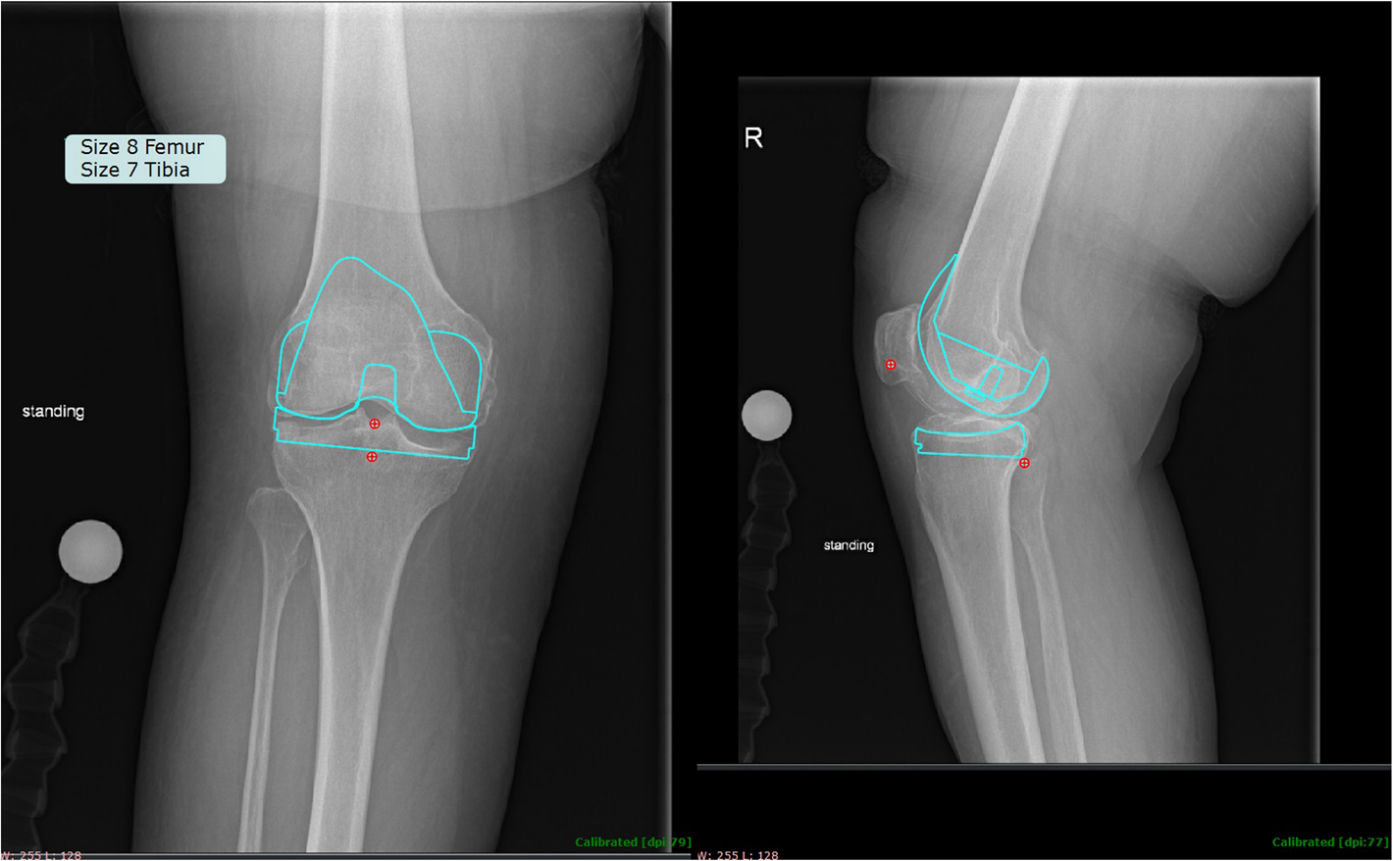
Example case where demographic information outperformed radiographic templating. Here the patient’s demographic information calculated a size-4 and size-3 femoral and tibial component, respectively; perfectly matching the sizes implanted. Meanwhile, radiographic templating showed good fit with a size-8 and size-7 femoral and tibial component, respectively
